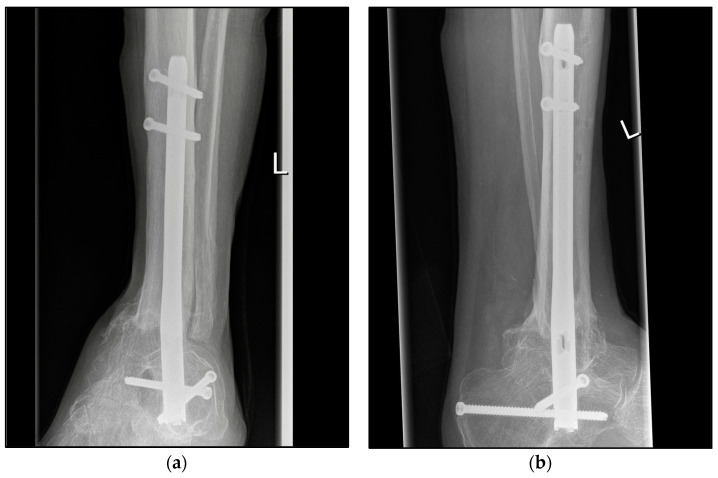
Postoperative radiographic findings of end-stage posttraumatic arthritis of the left ankle with septic history of a 82-year-old male treated with a tibiotalocalcaneal arthrodesis T2™ Ankle Arthrodesis Nail, 200 × 10 mm. (a,b) Anteroposterior and lateral view; view, 5 years post operation.
